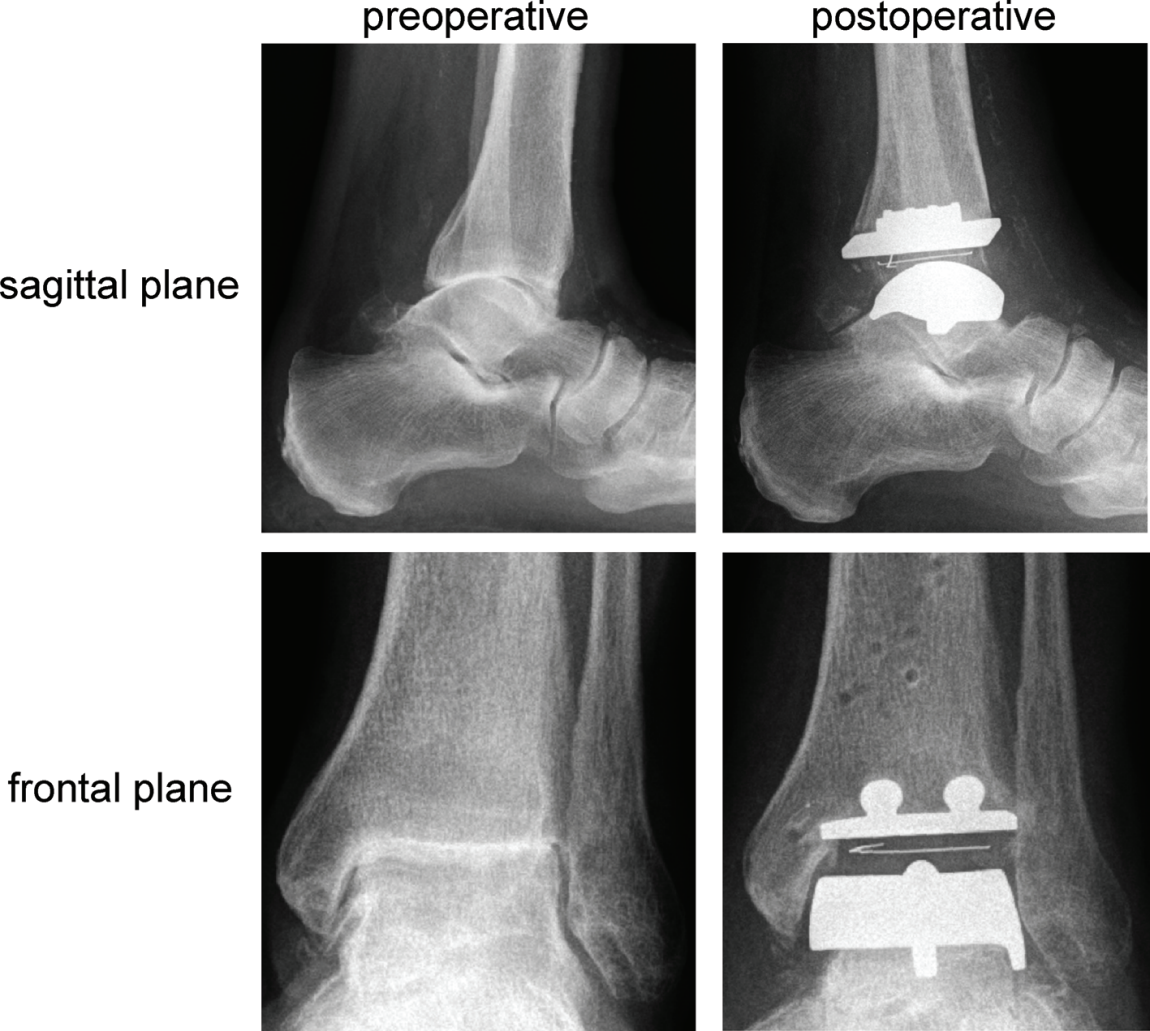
Radiographs of a left arthrotic ankle joint pre-and postoperative in sagittal and frontal plane.
